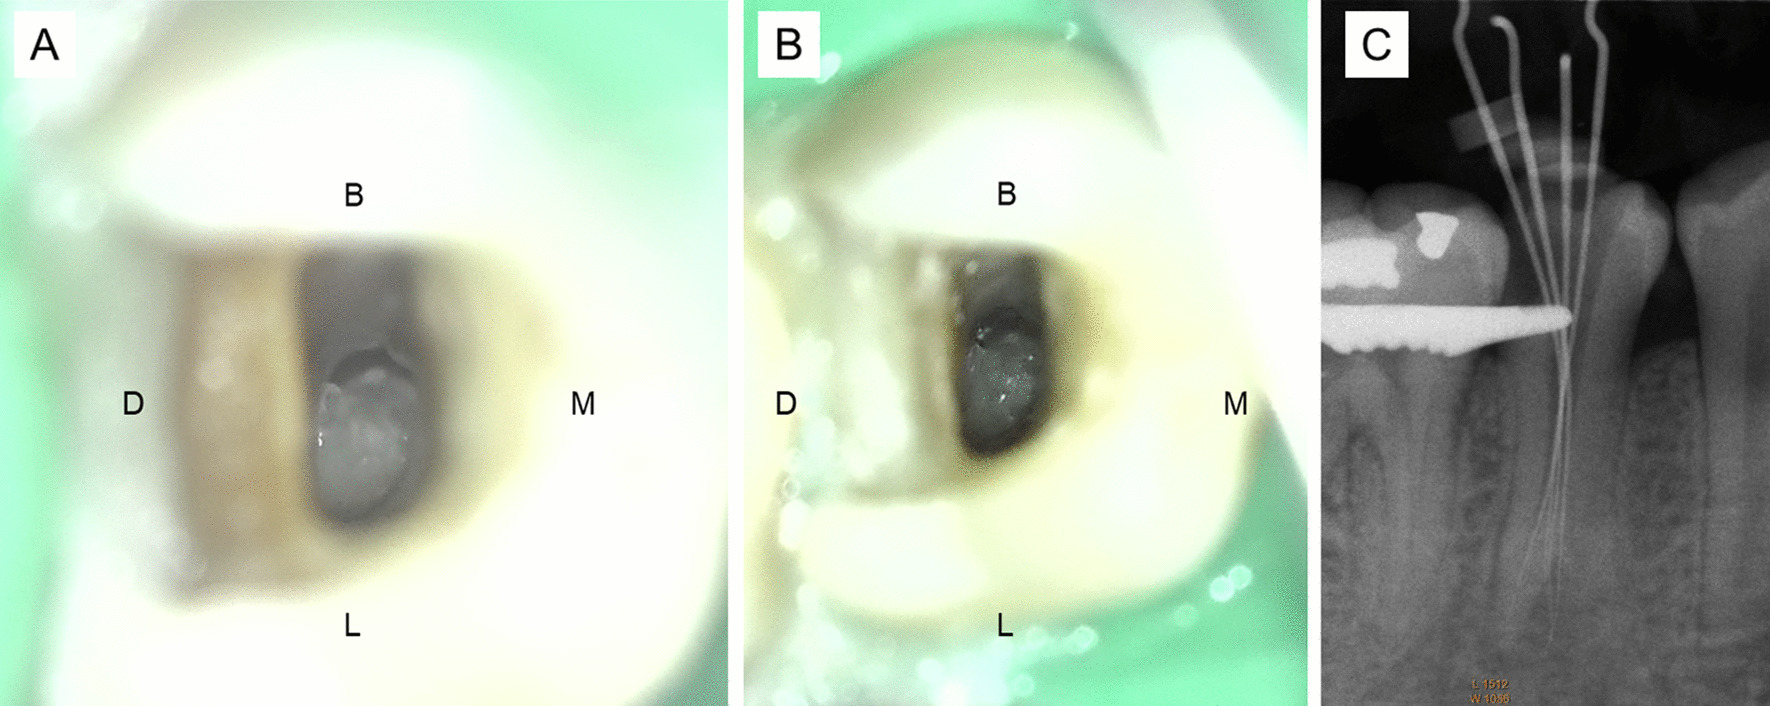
Right mandibular second premolar with a C-shaped canal configuration. A A semicolon shape (C2 category), B Two Krasner and Rankow laws can be applied in this tooth, Law of Color Change: the color of the pulp-chamber floor is always darker than the walls and Law of Orifice Location 1: the orifices of the root canals are always located at the junction of the walls and the floor C a radiograph with files inside the root canals from left to right are one file in the distolingual canal and 3 files in the buccal canal respectively. M, D, B, and L indicate mesial, distal, buccal and lingual aspect of the tooth respectively
